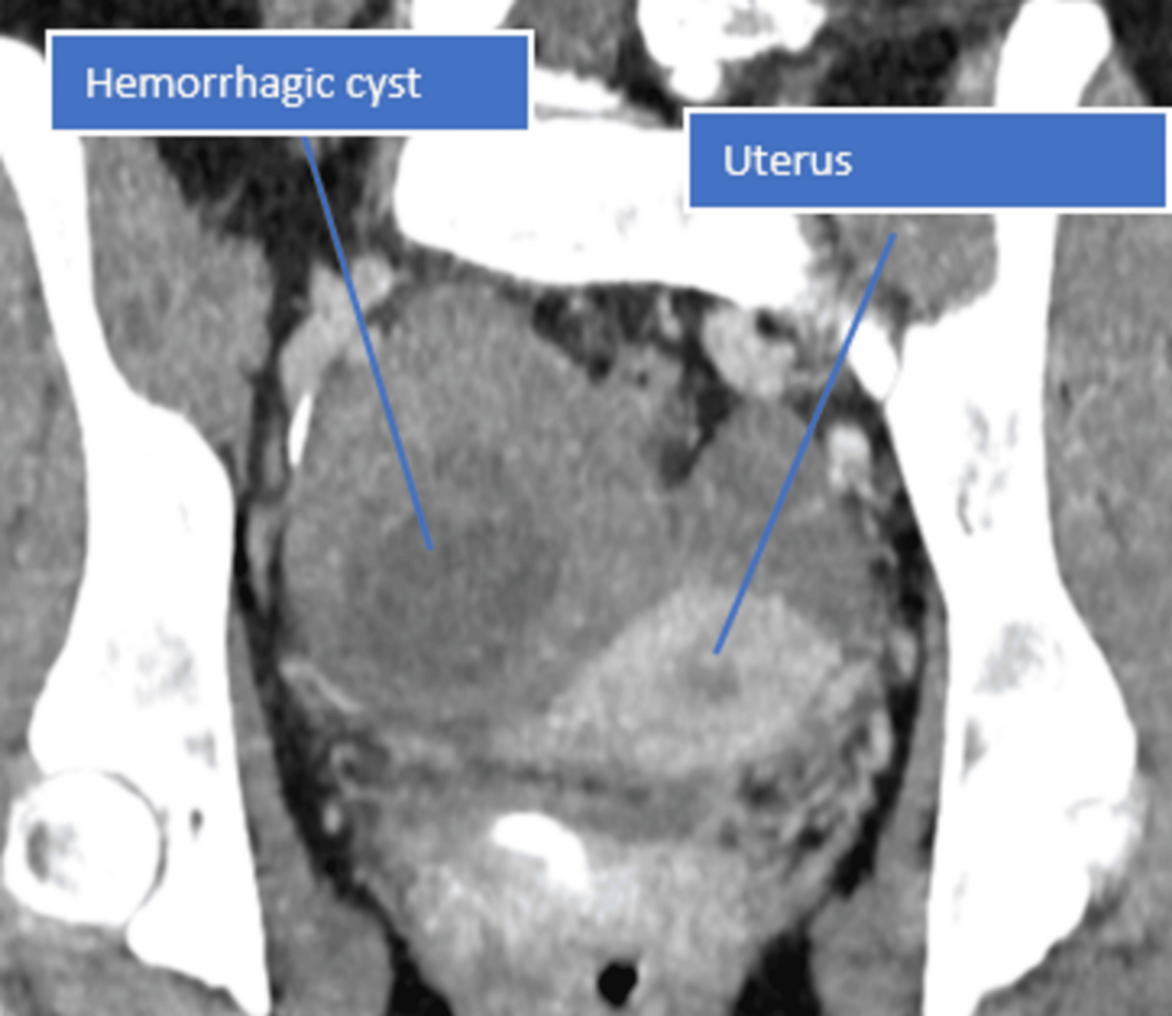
Longitudinal CT scan of the abdomen shows the haemorrhagic cyst
